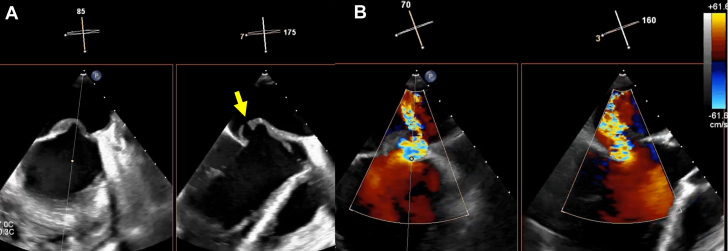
TEE and Color Doppler Imaging of the MV TTE (A) and color Doppler imaging (B) reveal a 3.8 mm perforation and thickening in the A2-A3 segment of the anterior mitral leaflet, accompanied by severe mitral regurgitation originating from the same site. MV = mitral valve; TEE = transesophageal echocardiogram; TTE = transthoracic echocardiogram.
